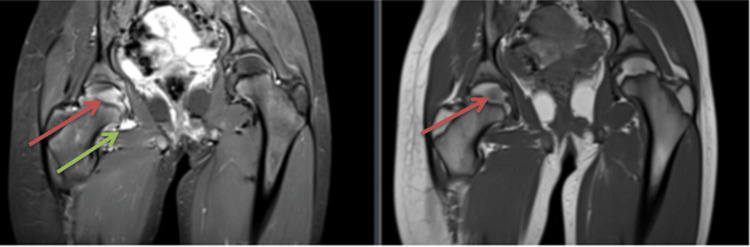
T2-weighted image with a fat-saturation sequence (left). T1-weighted image: coronal MRI hip (right) showing a band-like rectangular focal abnormal signal on the right femoral head, low on the T1-weighted image (red arrow on the right side) and bright on the T2-weighted image with a fat-saturation sequence (red arrow on the left side). Abnormal bright signal intensity is present on the T2-weighted image representing the right hip joint effusion (green arrow on the left).
